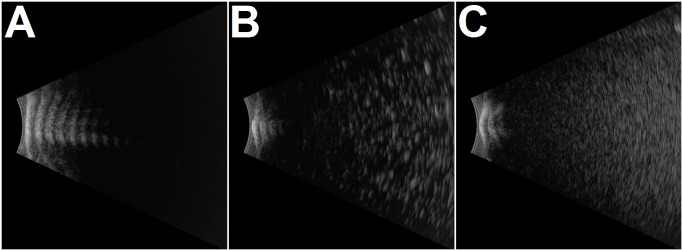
B-scan ultrasound images obtained after visualising (A) liposomes, (B) conventional nanobubbles and (C) optimised nanobubbles using diagnostic ultrasound.
